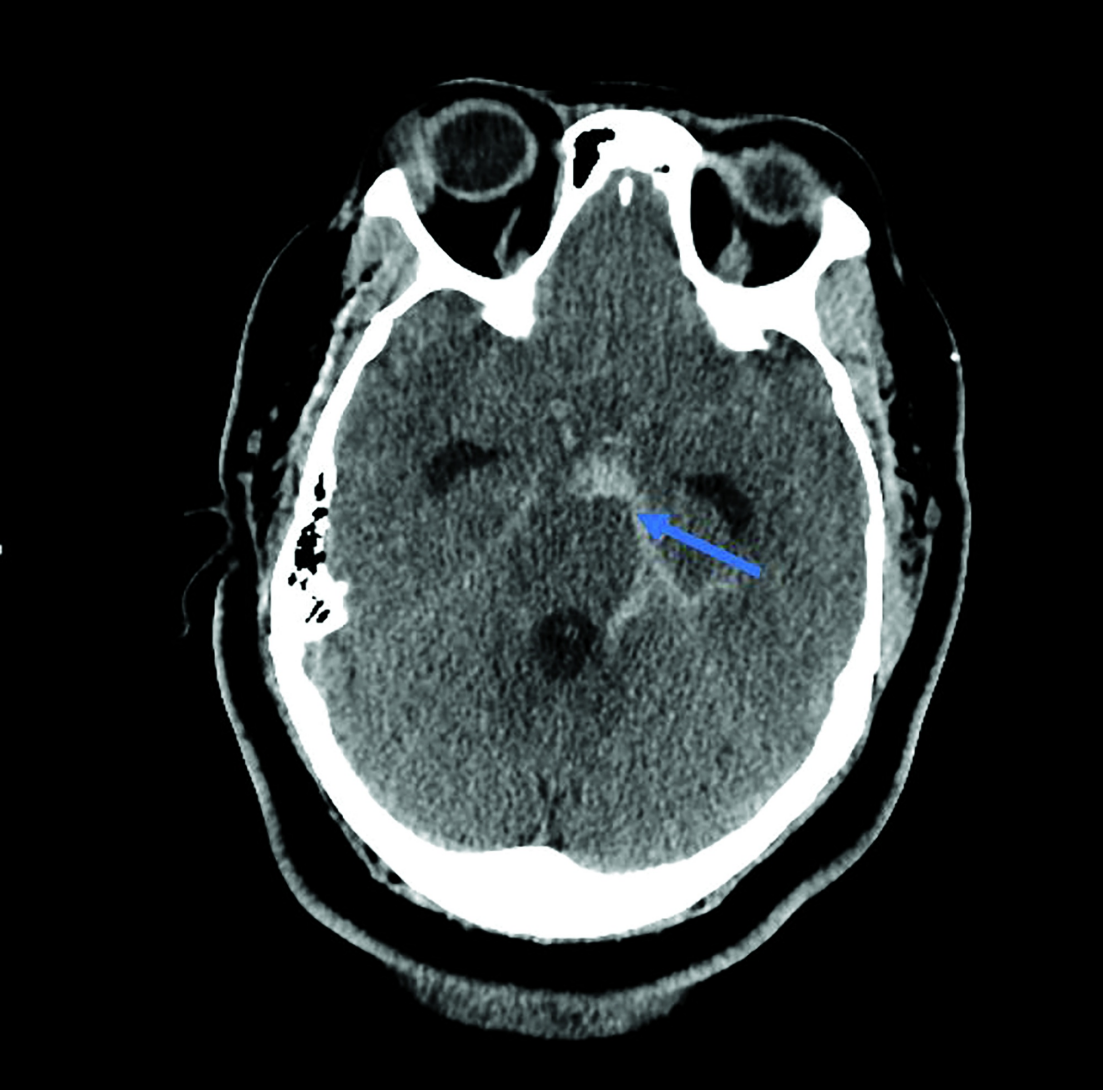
Computed tomography of the head Computed tomography (CT) of the head demonstrating asymmetric subarachnoid hemorrhage in the left ambient and quadrigeminal cisterns (blue arrow) with early hydrocephalus.
